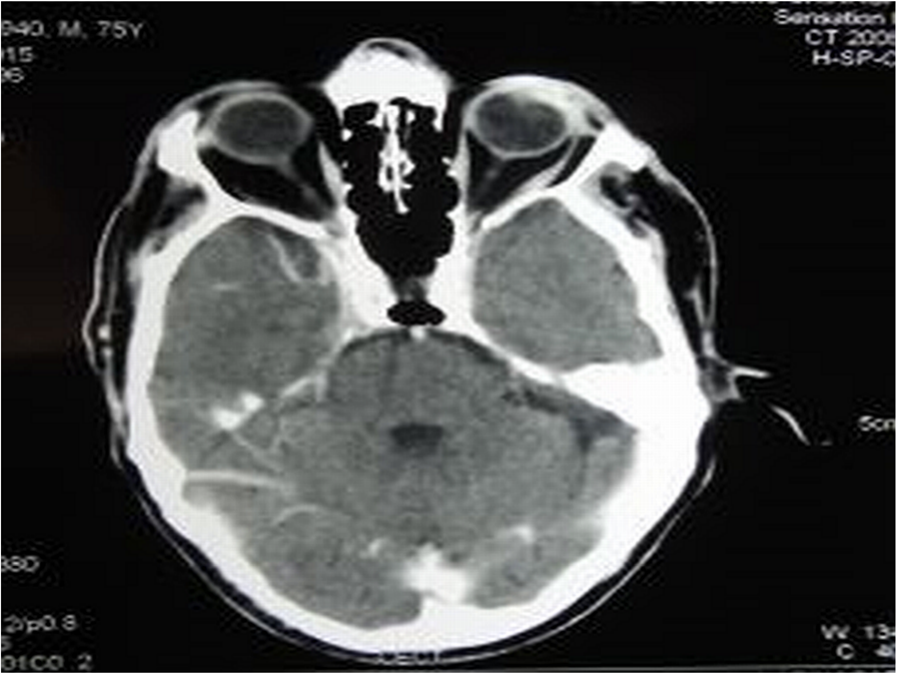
Preoperative contrast enhanced computed tomography of patient showing enhancing lesion involving anterolateral wall of the left eyeball and thickened overlying eyelid
